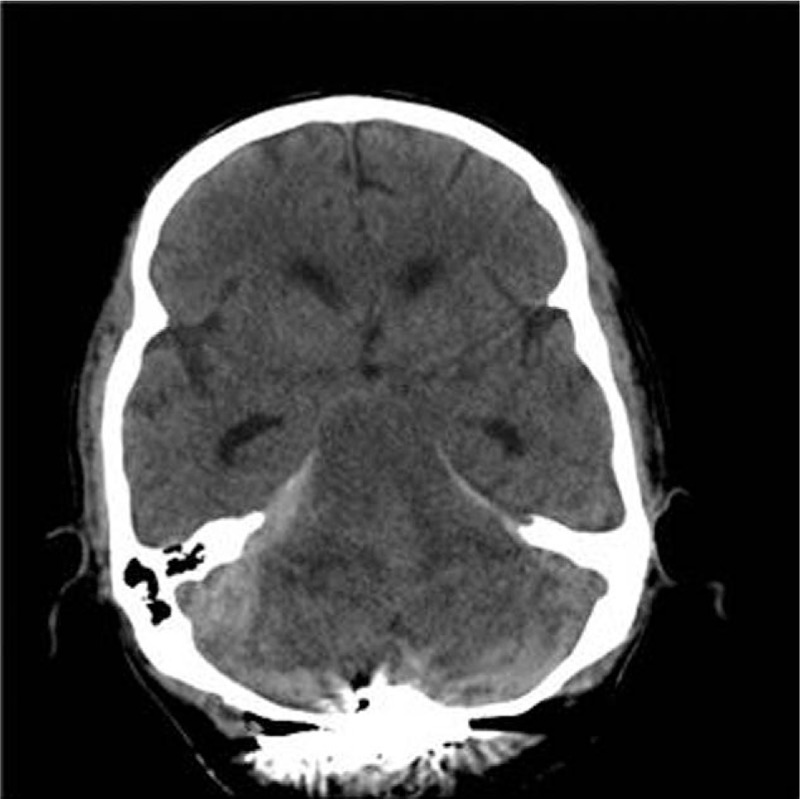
Postoperative brain computed tomography image of the patient showing infratentorial hemorrhage along tentorium cerebelli.
